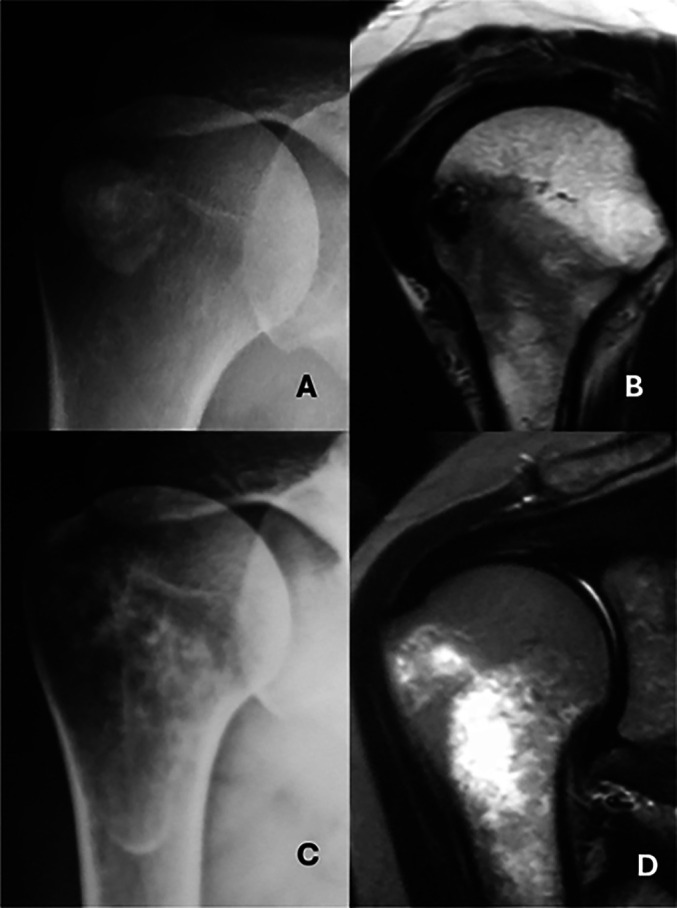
A. AP view showing a calcification located in the tendon of the infraspinatus muscle. B. Oblique sagittal MRI view showing the calcification and the intraosseous deposit. C. Anteroposterior (AP) radiographic image showing extension to the medullary canal of the humerus D. MRI coronal view highlights intramedullary extension.
